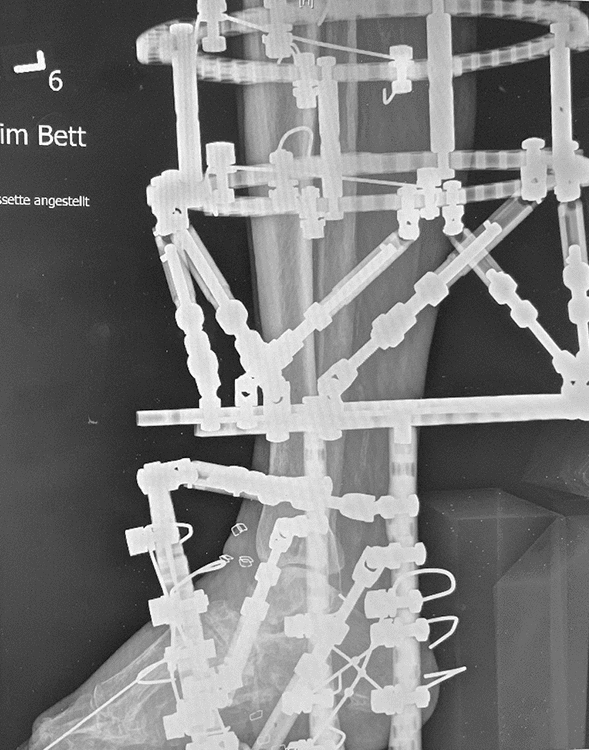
Lateral x-ray shows attached TSF for simultaneous arthrodesis in tibiotalar und subtalar joints
